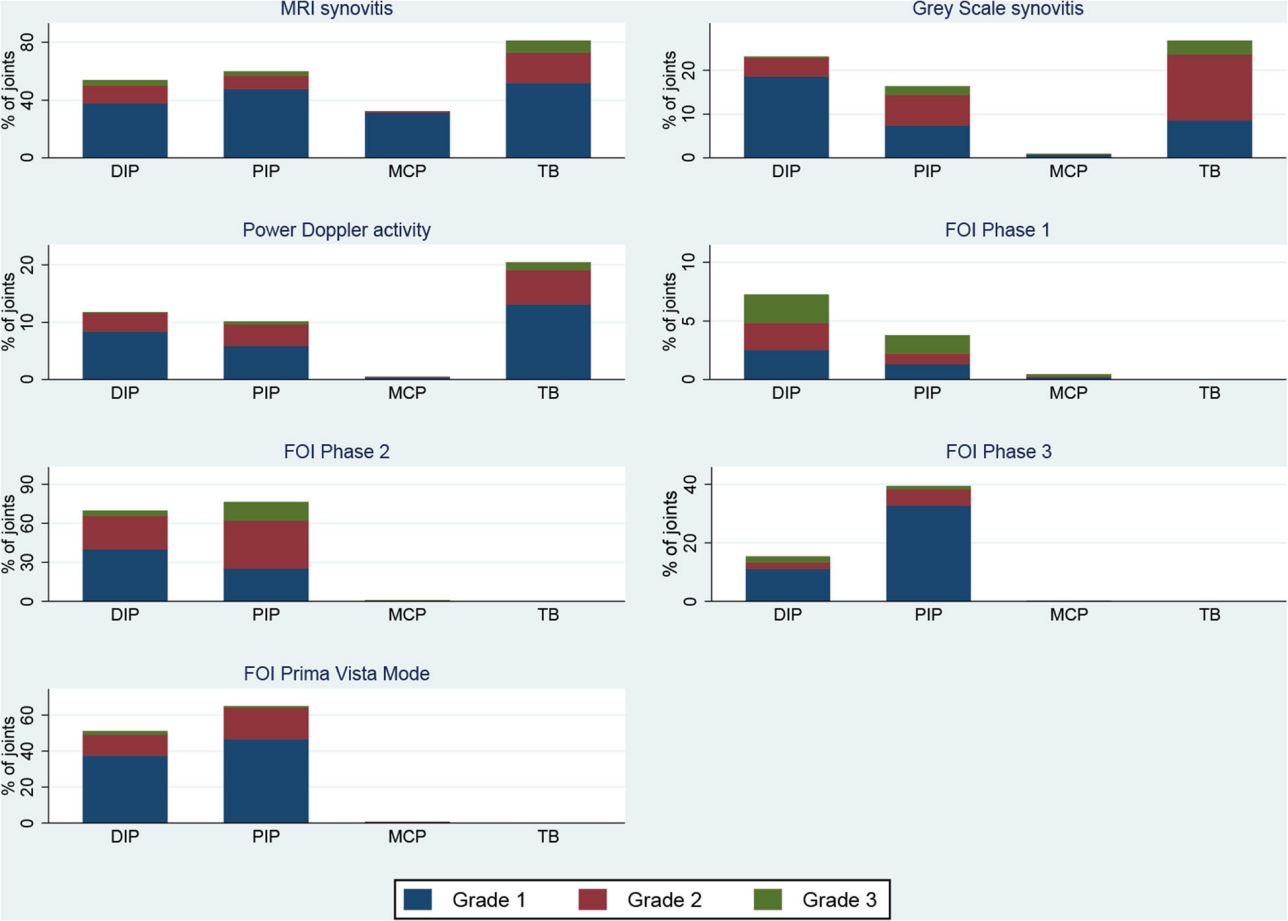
Frequency distribution of FOI enhancement, MRI and gray-scale synovitis and power Doppler activity in hand OA patients. MRI, magnetic resonance imaging; DIP, distal interphalangeal joints; PIP, proximal interphalangeal joints; MCP, metacarpophalangeal joints; TB, thumb base. ^1^MRI findings from dominant hand only, FOI and ultrasound from bilateral hands.^2^The thumb base (TB) includes CMC-1 and/or STT synovitis for MRI and CMC-1 synovitis for ultrasound. The TB region is assessed as a whole for FOI, as the CMC-1 and STT joint cannot be distinguished
